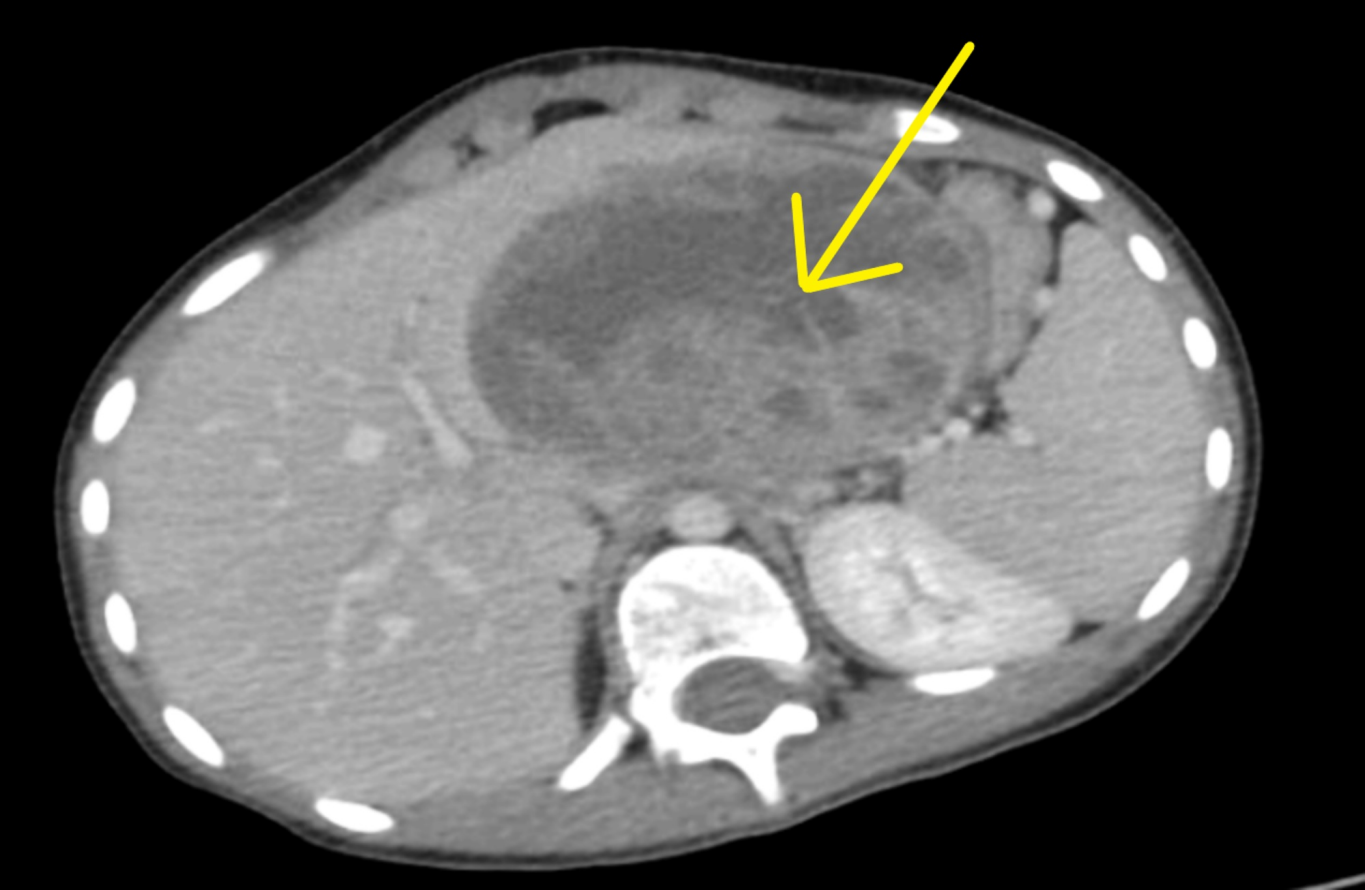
CT scan showing a huge lesion measuring 6.2 x 8.7 x 9.4 in diameter.
